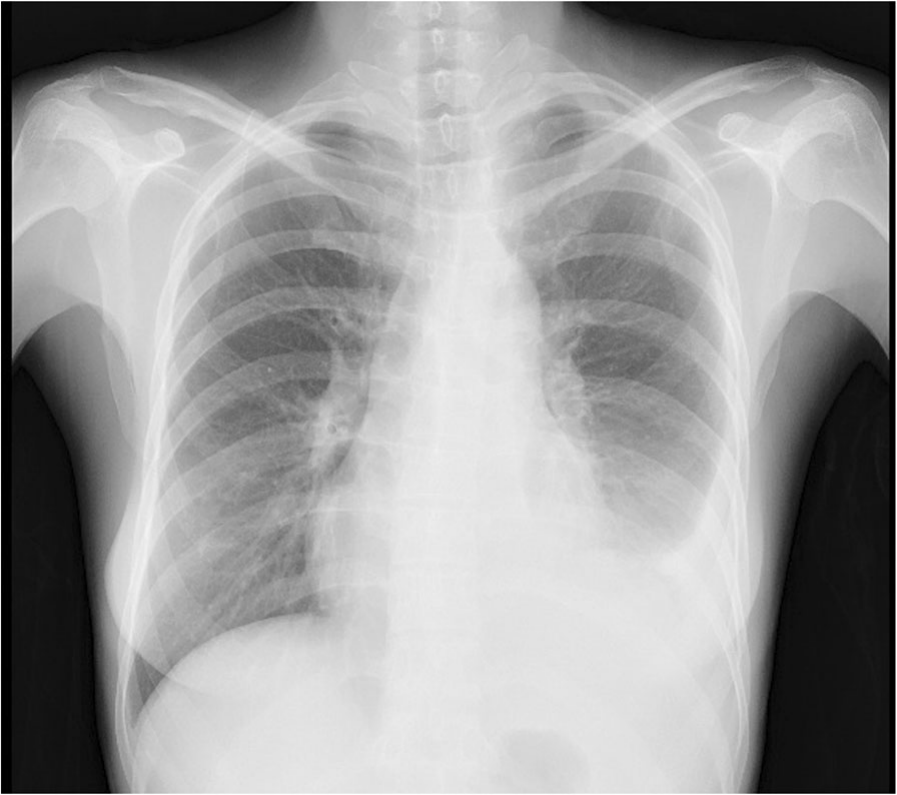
Chest CT shows pleural loculated effusions in the left lung
